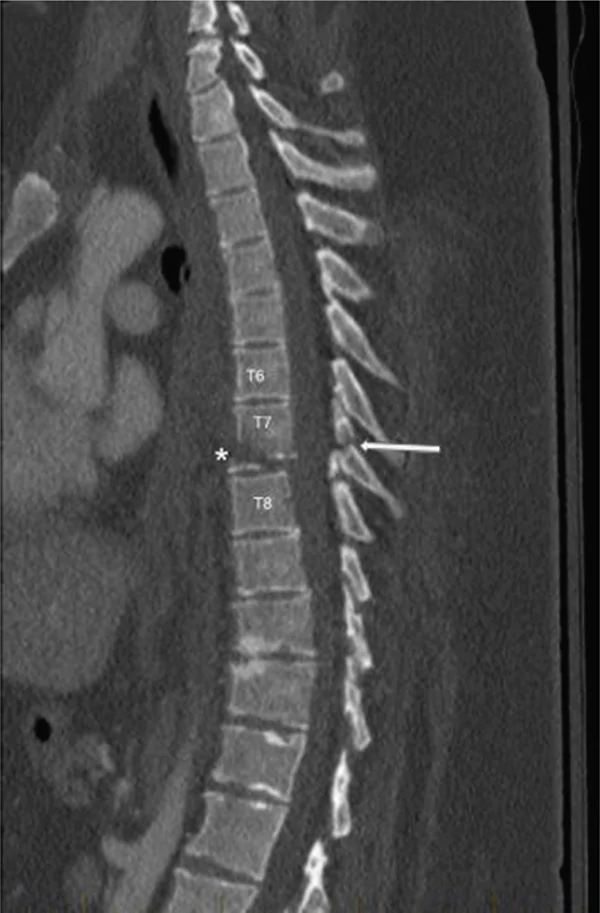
Sagittal thoracic spine computed tomography demonstrates acute displaced fractures involving the inferior endplate ( ^∗^) and the right lamina of T7 (←). Additional fracture of the left T7 lamina is not shown here. Grade I retrolisthesis of T7 on T8 is also noted with up to 7 mm of narrowing of the spinal canal.
